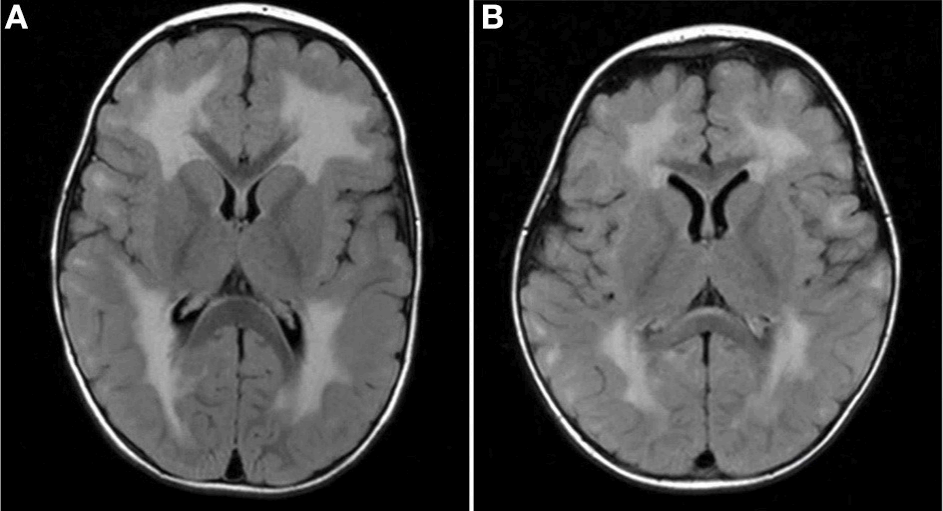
MRI findings in MDC1A-patients included in the study. Brain MRI TIRM (Turbo-Inversion Recovery-Magnitude, axial) show diffuse symmetrical white matter lesions predominantly in the periventricular regions and no involvement of thalamus or brainstem in both cases. (A) Patient 3 with expanded supratentorial leukencephalopathy at two years of age. (B) Patient 4 diffuse leukencephalopathy.
